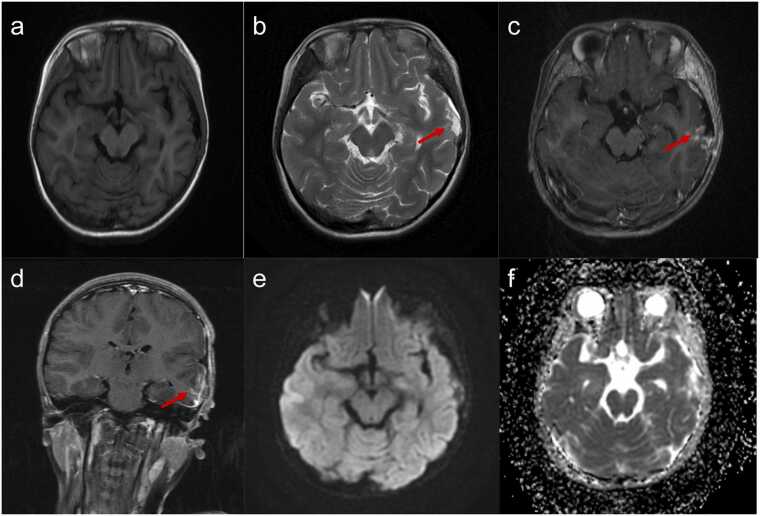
Cranial magnetic resonance imaging 1 year after proton beam therapy. (a) Axial T1WI and (b) T2WI: The postsurgical cavity has narrowed. (c) Axial and (d) coronal T1 postcontrast images: The ring-like enhancement around the lesion has significantly weakened, becoming spotty. (e) Diffusion-weighted imaging: The lesion signal has changed from hyperintense to hypointense. (f) Apparent diffusion coefficient maps: The lesion exhibited increased signal in apparent diffusion coefficient maps.
